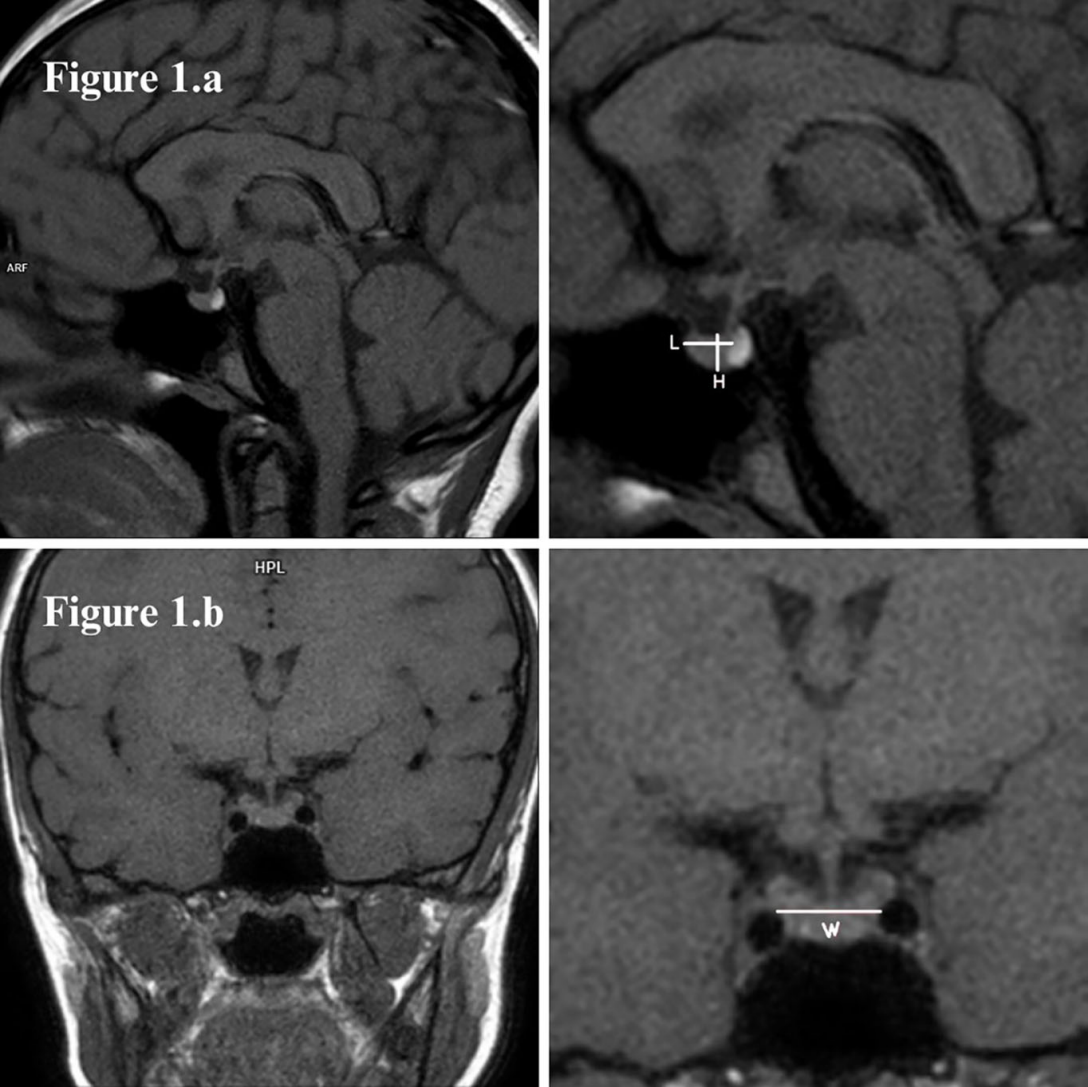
(A) Sagittal and (B) coronal T1-weighted MR images of a normal size pituitary gland, with bright spot of the posterior lobe (neurohypophysis) normally located and well-centered pituitary stalk (infundibulum). Pituitary measures were taken as shown: length (L) and height (H) in sagittal images, width (W) in coronal.
